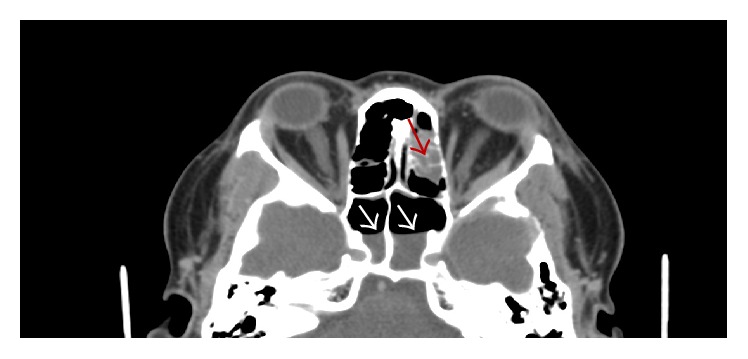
CT with contrast in the sinuses demonstrates opacification of certain ethmoid cells (red arrow) and fluid level in the sphenoid sinus bilaterally (white arrow).
